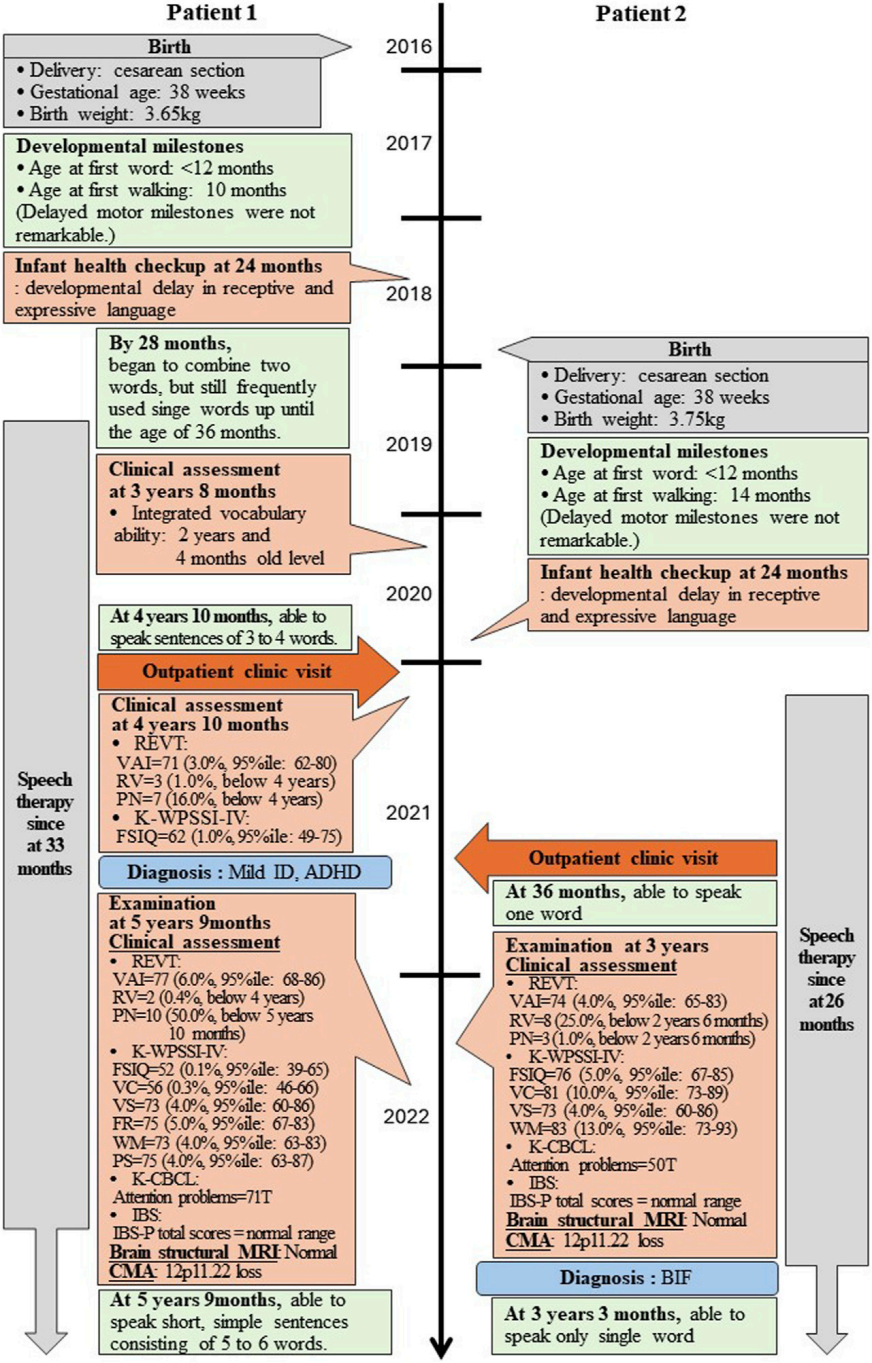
Timeline of two patients’ assessment and outcomes. *REVT*: receptive and expressive vocabulary test; *VAI*: vocabulary ability index; *RV*: receptive vocabulary; *PN*: picture naming; *K-WPSSI-IV*: Korean Wechsler preschool and primary scale of intelligence fourth edition; *FSIQ*: full scale intelligence quotient; *VC*: vocabulary comprehensive; VS: visual spatial; *FR*: fluid reasoning; *WM*: working memory; *PS*: processing speed; *CBCL*: child behavior checklist; *IBS*: interactive behavioral scale; *MRI*: magnetic resonance imaging; *CMA*: chromosomal microarray analysis; *ID*: intellectual disability; *BIF*: borderline intellectual functioning; *ADHD*: attention deficit hyperactivity disorder.
